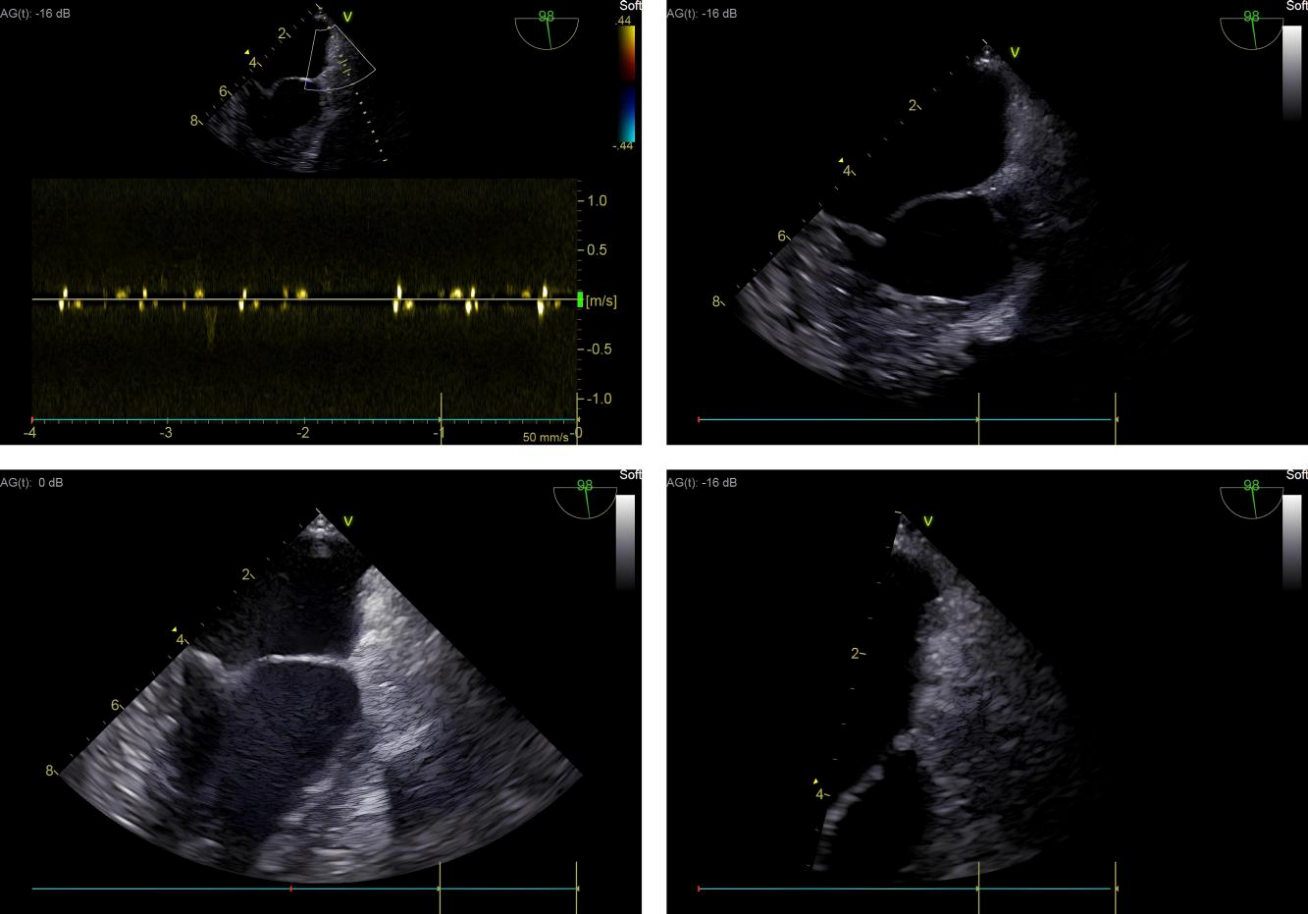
Intraoperative transoesophageal echocardiography with no Doppler flow in the region where the left atrial appendage would be located.
